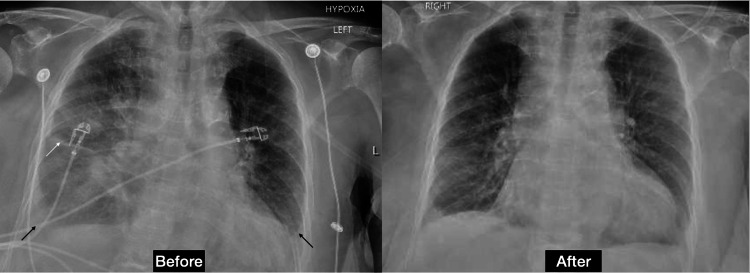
Chest X-ray before and after the first thoracentesis Worsening pleural effusion predominantly on the right side (black arrow) and Kerley line (white arrow).
